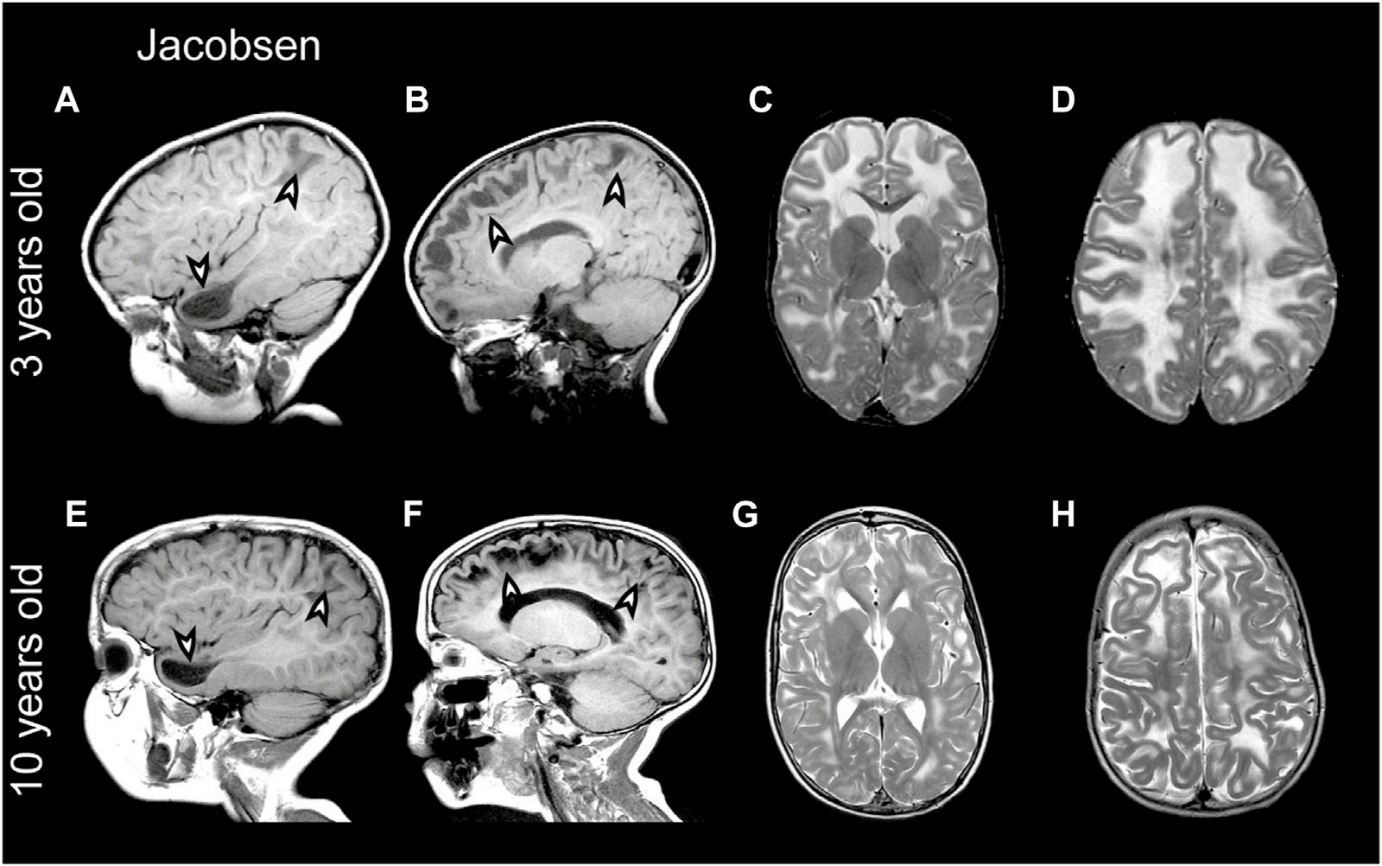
MRI findings in a Jacobsen syndrome patient. (A–D) MRIs from a 3-year-old Jacobsen syndrome patient with a chromosomal deletion that includes *GLIALCAM*. Numerous anterior temporal and frontal subcortical cysts are visible in the sagittal T1-weighted MRIs in panels A and B (arrowheads). T2-weighted images in (C and D) reveal extensive signal abnormality and swelling of the cerebral white matter. (E–H) Follow-up of the same patient at 10 years of age. Subcortical cysts persist [arrowheads in (E,F)], but there is clear improvement in signal abnormality and swelling of the cerebral white matter.
